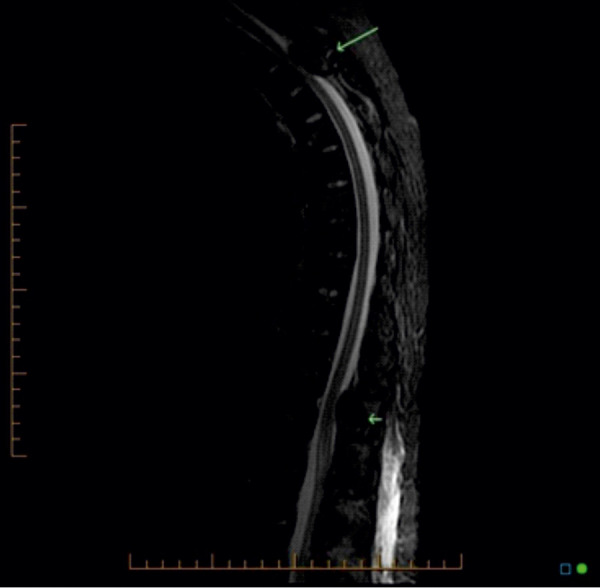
MRI of thoracic spine: Severe spinal canal stenosis and cord edema at T3, moderate stenosis at T12.
